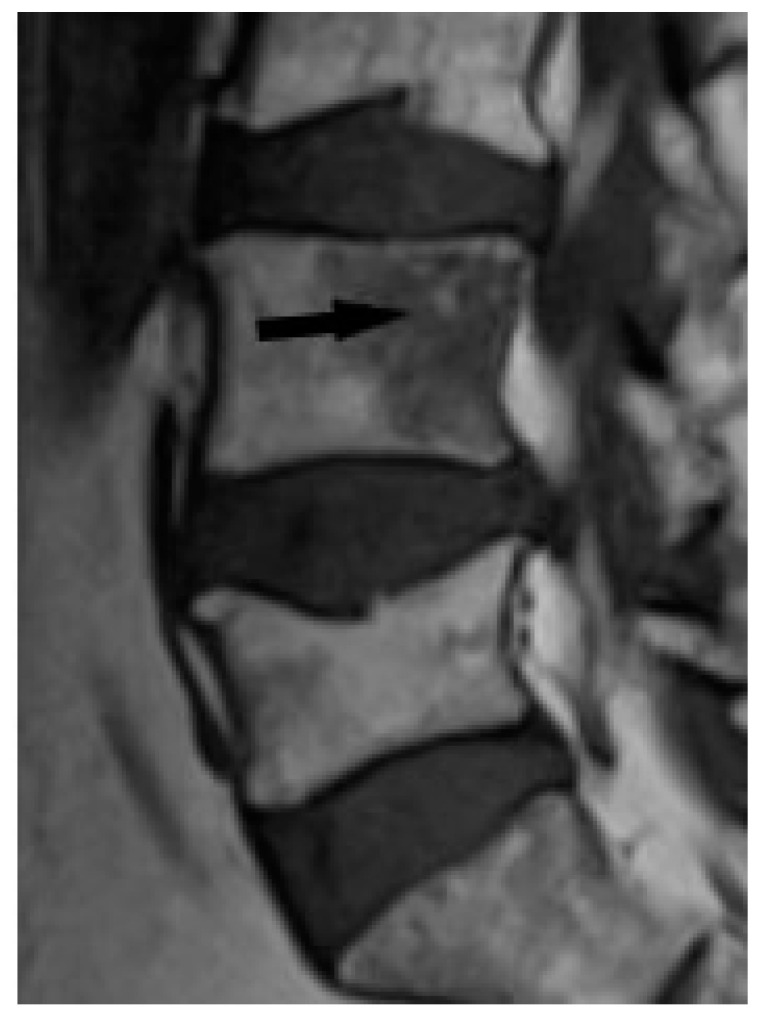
T1-weighted image. Hypointense lesion with irregular margins and with small foci of hyperintense fat (black arrow).
